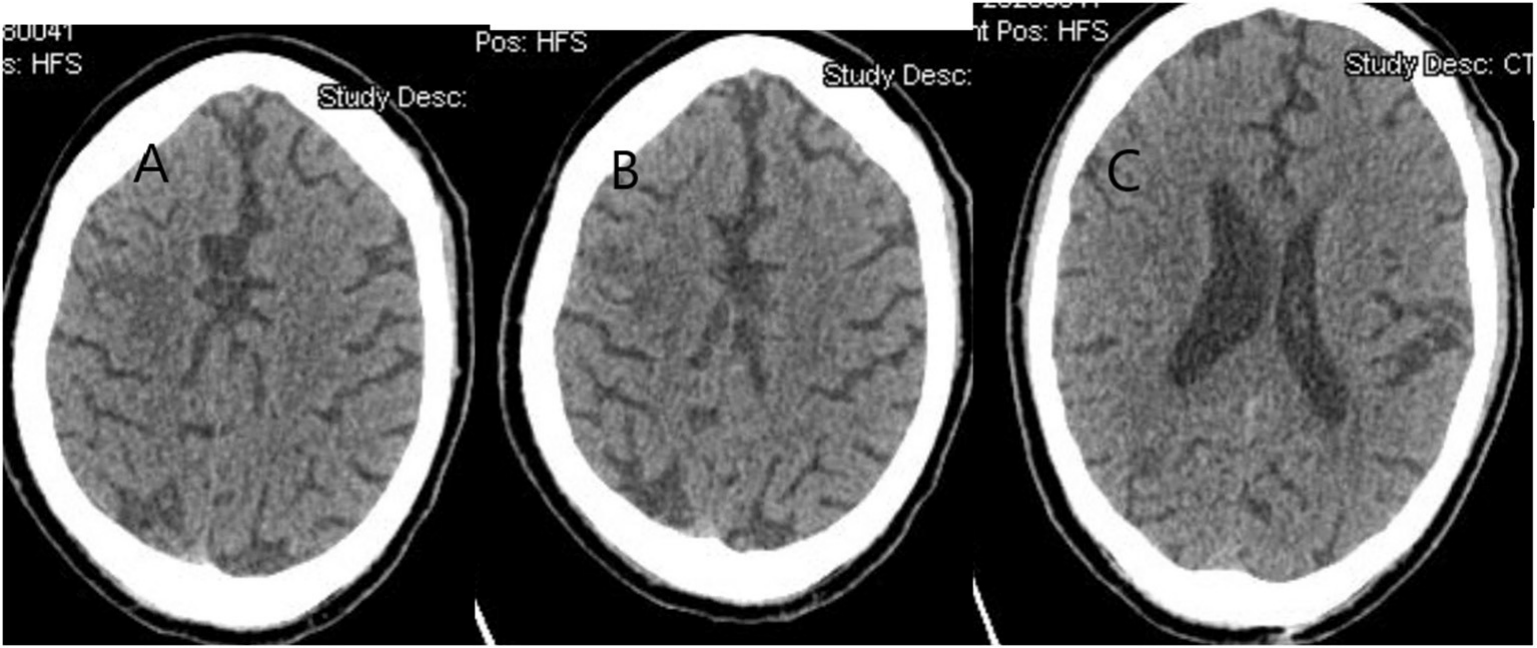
A 68-year-old man complained of acute weakness of the left side of her body 3 days after being diagnosed with COVID-19. Brain CT without contrast shows multiple foci of infarcts in the right middle cerebral artery involving the right frontal, parietal, and temporo-occipital junction.
